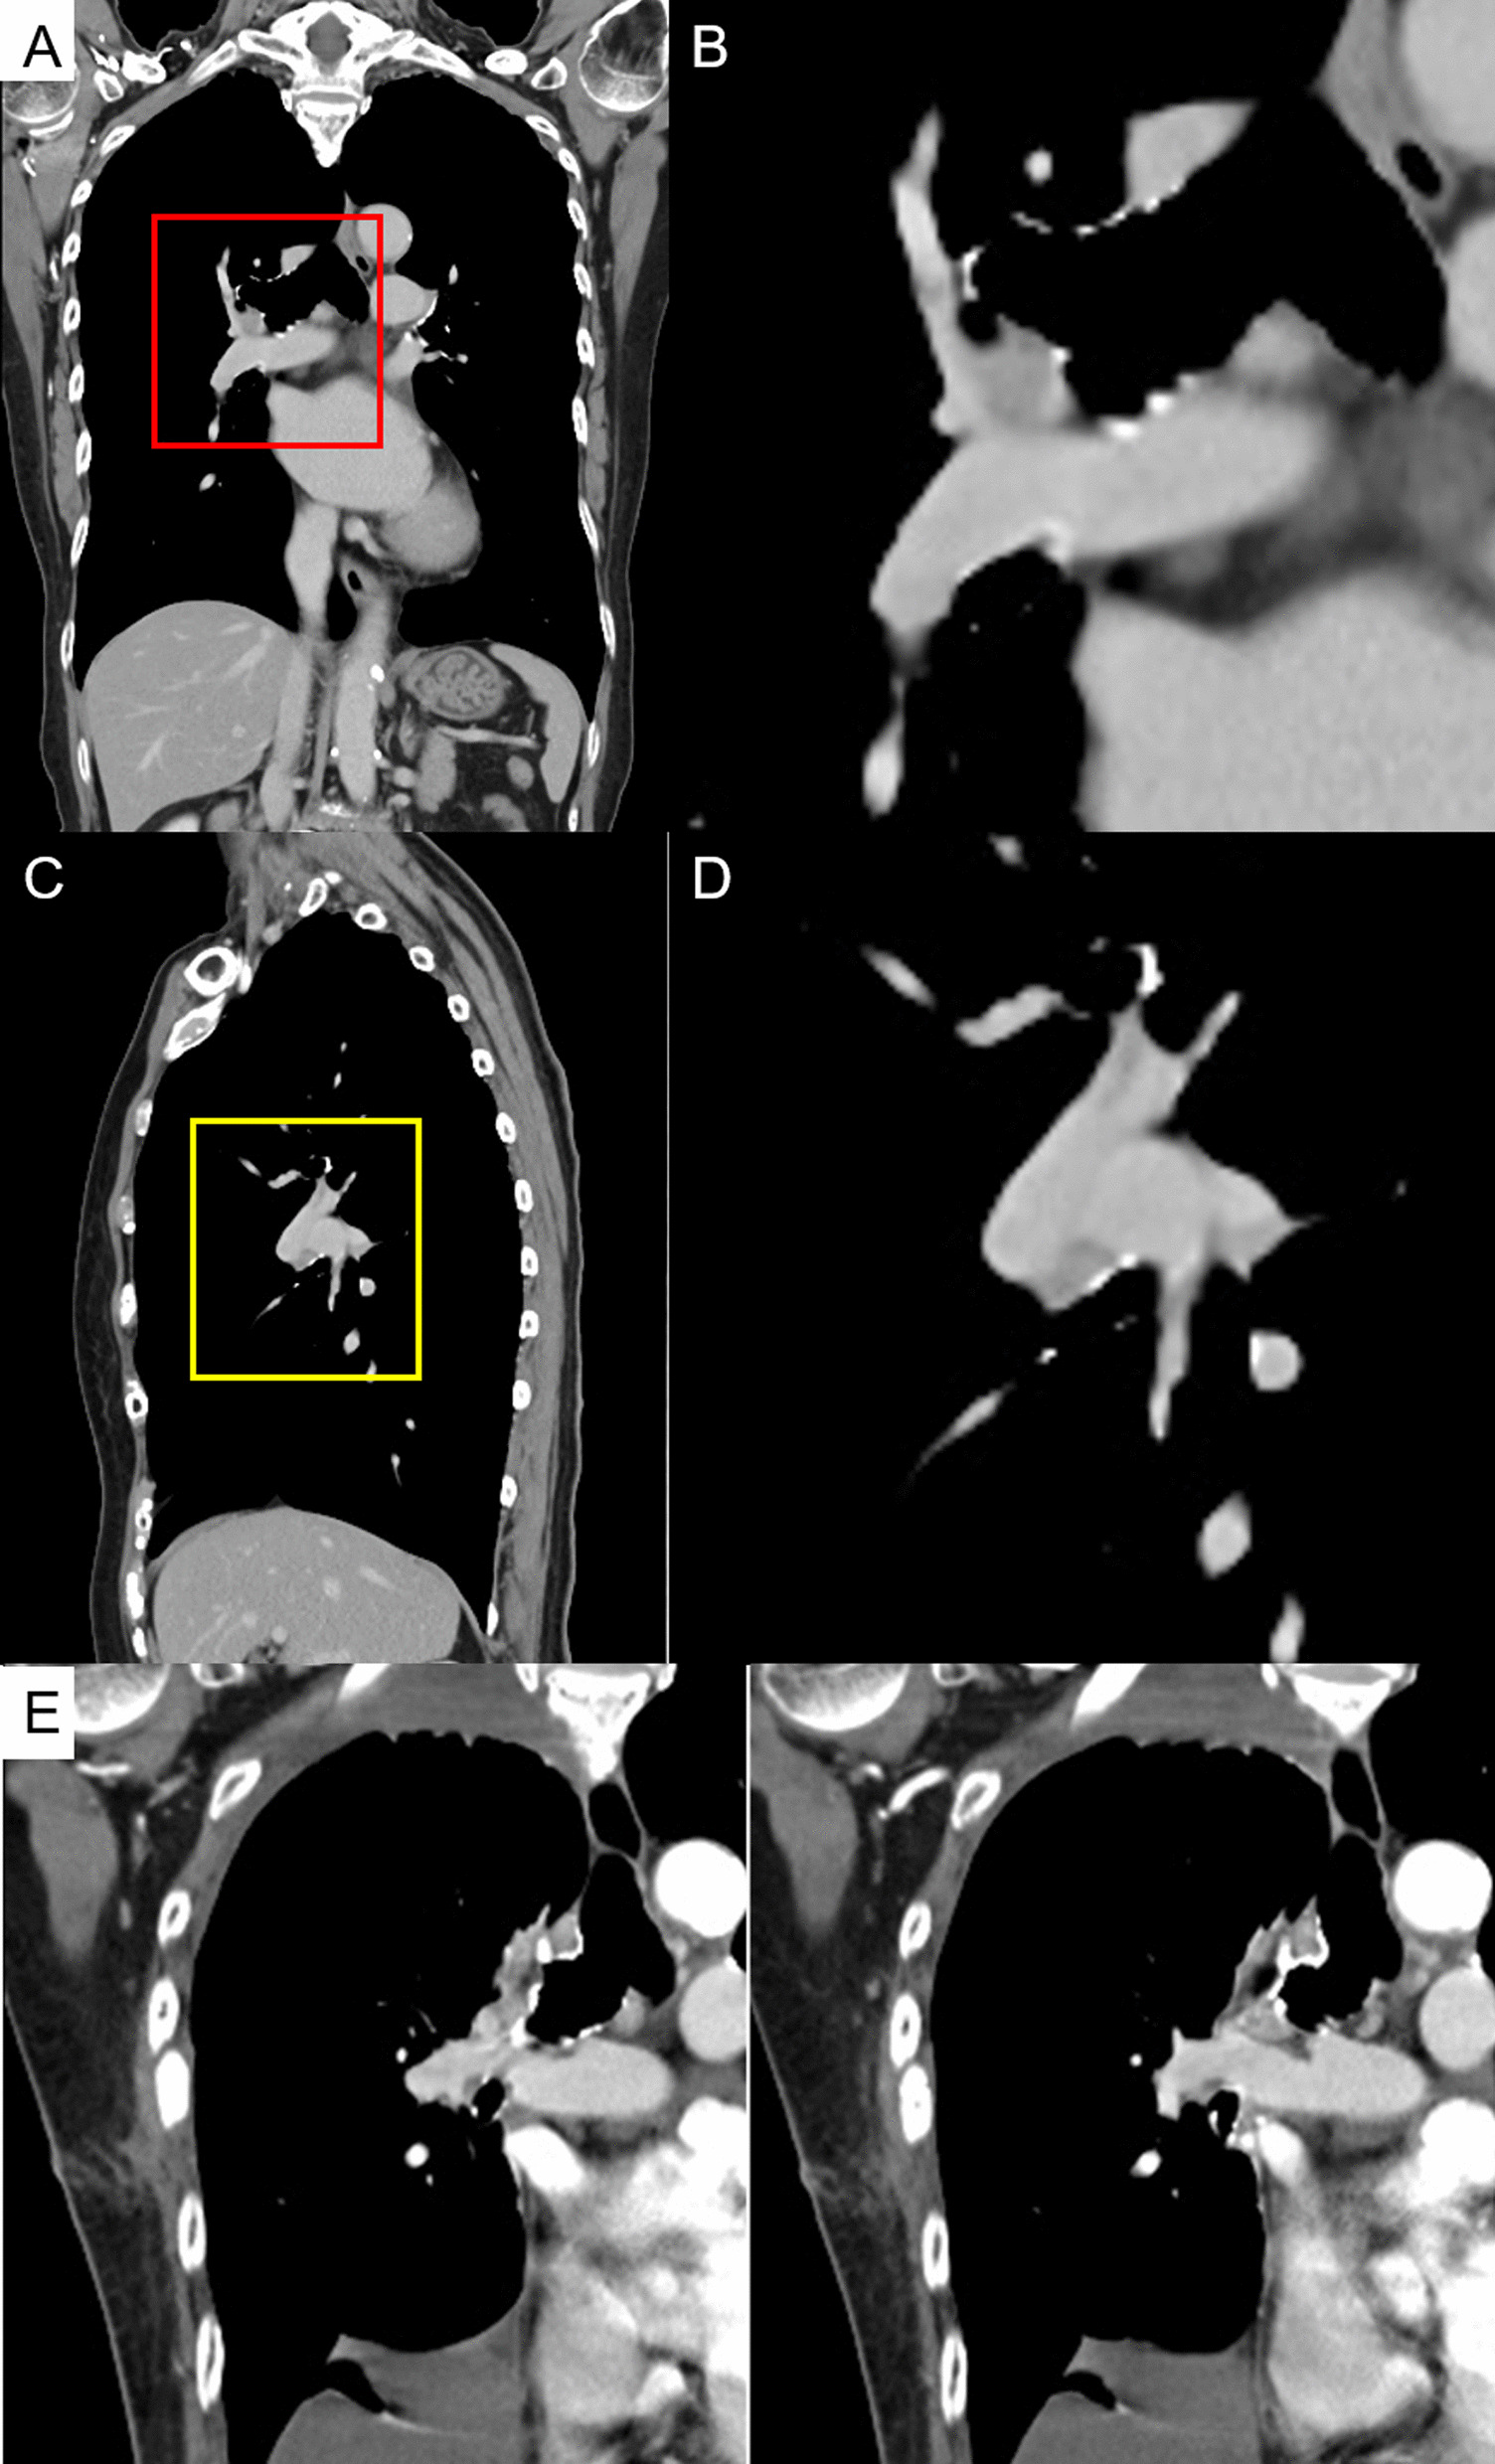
Chest contrast-enhanced computed tomography showing inflammatory lymph node infiltration of the hilar lymph node. A, B show the coronal views. C, D show the sagittal view. B is in the same range as the red square in (A). D is in the same range as the yellow square in (C). E Postoperative chest contrast-enhanced computed tomography showing no aneurysm in the A2 treated area
